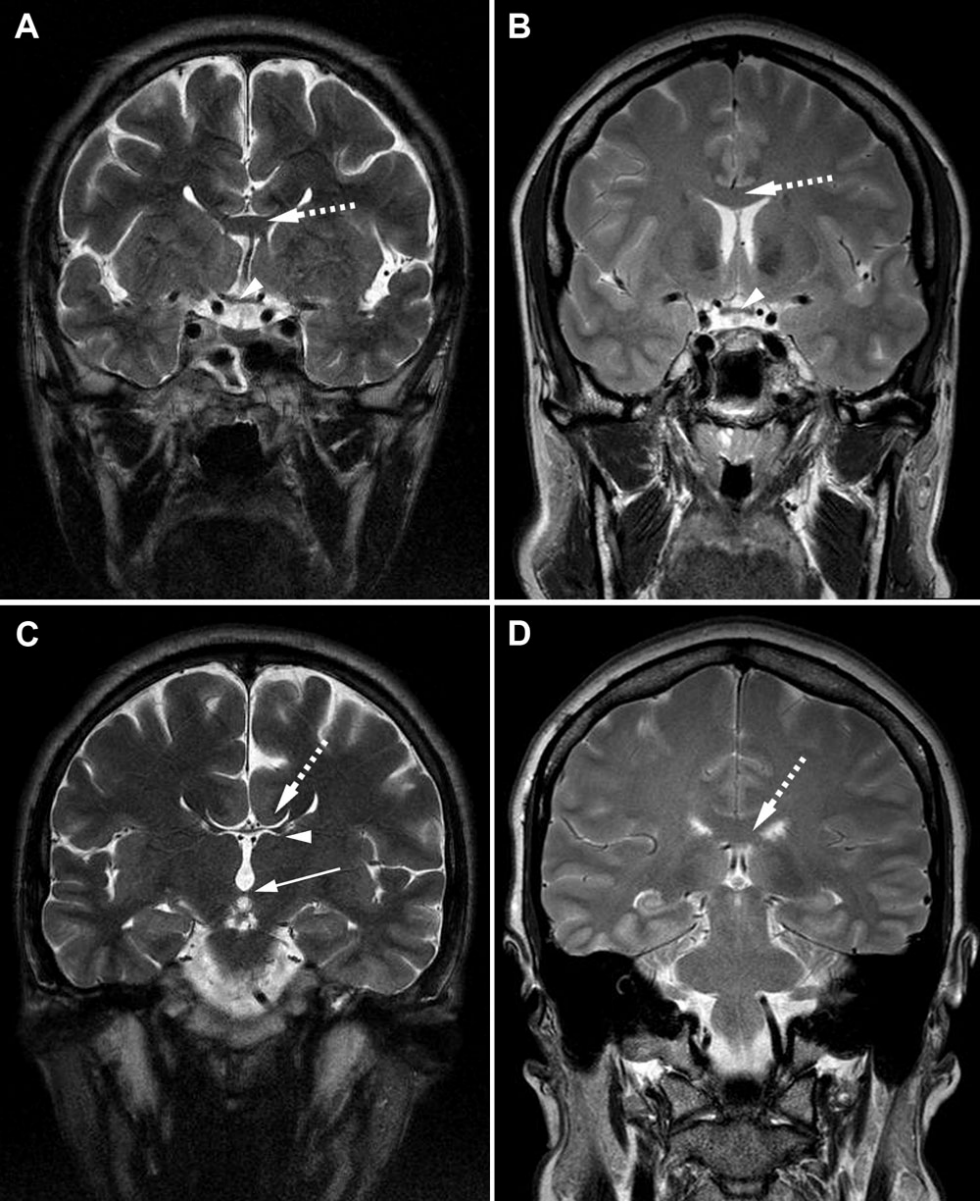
Coronal cerebral T2-weighted magnetic resonance images. A: Patient I-1 from Family 3. Dashed arrow shows severe hypogenesis of the corpus callosum with small amount of remnant tissue localized at the virtual connection between the genu and the body of the corpus callosum; arrow head shows the atrophic optic chiasm. B: Normal MRI images. Dashed arrow shows normal corpus callosum and arrow head shows normal optic chiasm. C: Patient I-1 from Family 3. Dashed arrow shows lateral callosal bundles of Probst, which are hemispheric connection fibers that did not cross the midline and that are seen in callosal dysgenesis. Superomedial margins of the lateral ventricles are indented by the Probst bundles. Arrow head shows remnants of the corpus callosum. Lower arrow shows normal posterior commissure. D: Normal MRI image with dashed arrow pointing normal corpus callosum.
